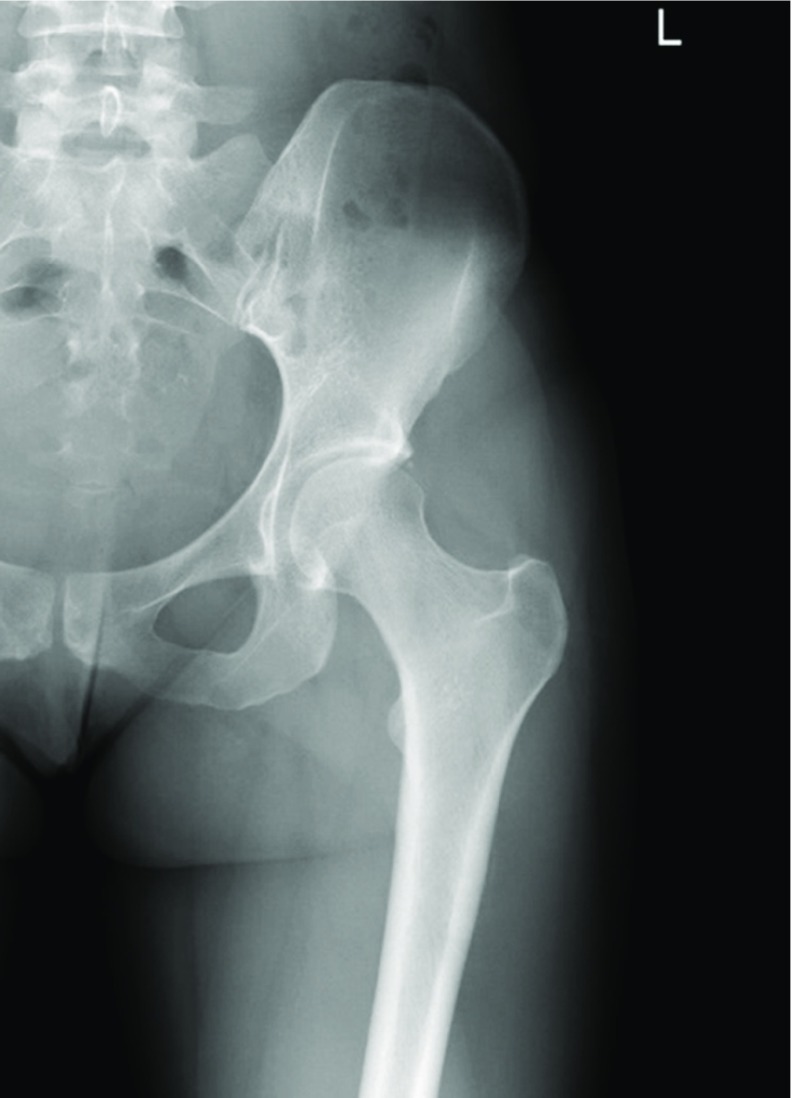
X-ray of the left hip (AP view), performed one month after the onset of the symptoms, which shows no signs of fracture.
